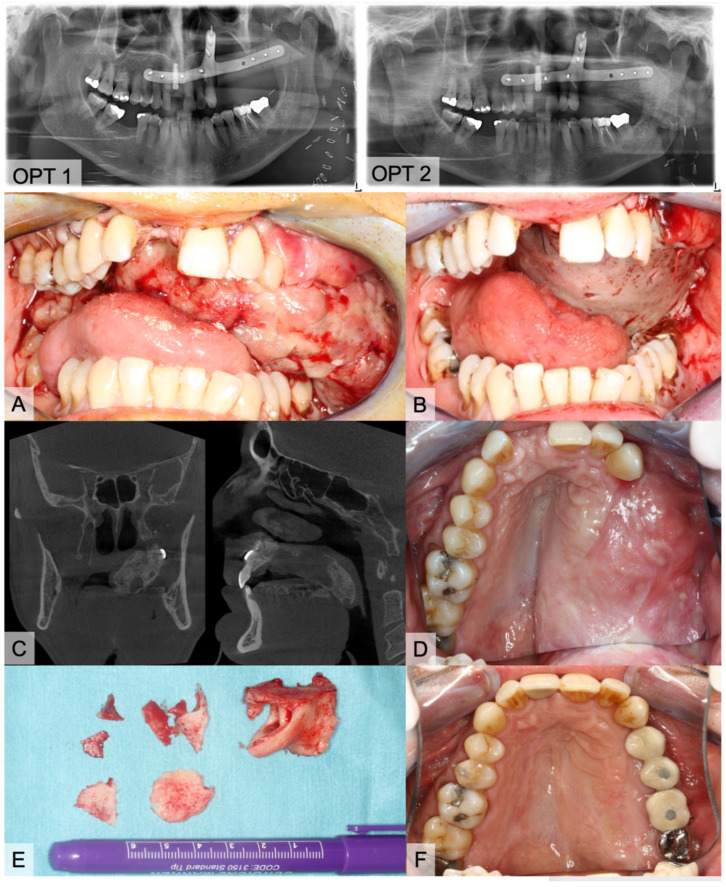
Images of an 54-year-old, healthy male who underwent subtotal hemi-maxillectomy due to a second recurrence of an odontogenic kerato-cyst (OKC). After more than one year later, we performed a virtually planned mono-segmental FFF transfer without a skin paddle for maxillary reconstruction (OPT 1). Three days after discharge, he consulted the emergency unit with progressive dyspnea and fear of asphyxiation in dorsal position. Clinical inspection showed large masses of soft and vulnerable granulation tissue (A). After carefully removing the tissue, we covered the bone with a split skin graft from the thigh (B). Six months later, we planned implantological rehabilitation. OPT and CBCT were accomplished and presented large bone masses at the palate (C, OPT 2). Clinical impression is shown on image (D). Prior to the insertion of four dental implants, we molded the FFF and removed bone masses (E). Implants were exposed four months later. Figure (F) shows the final prosthetic rehabilitation.
